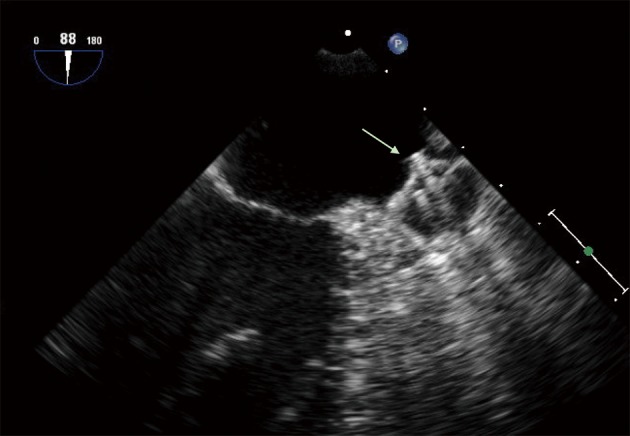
Transoesophageal echo view showing a Watchmann® device (arrow) filling the LAA lumen.
